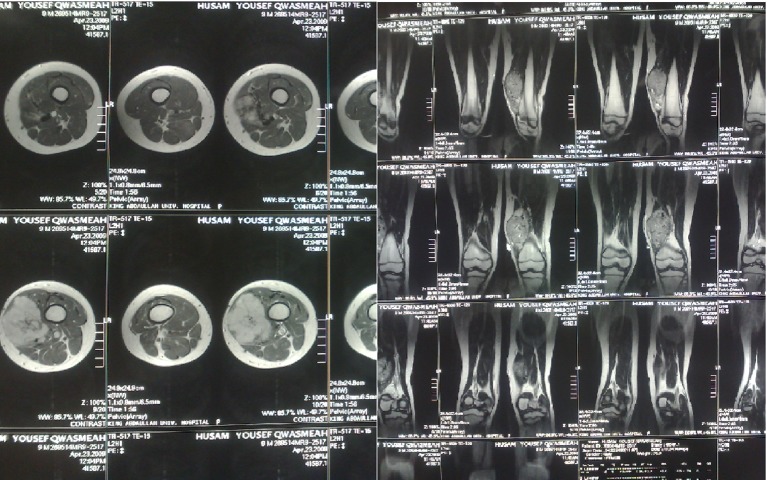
Pre-operative MRI findings.
